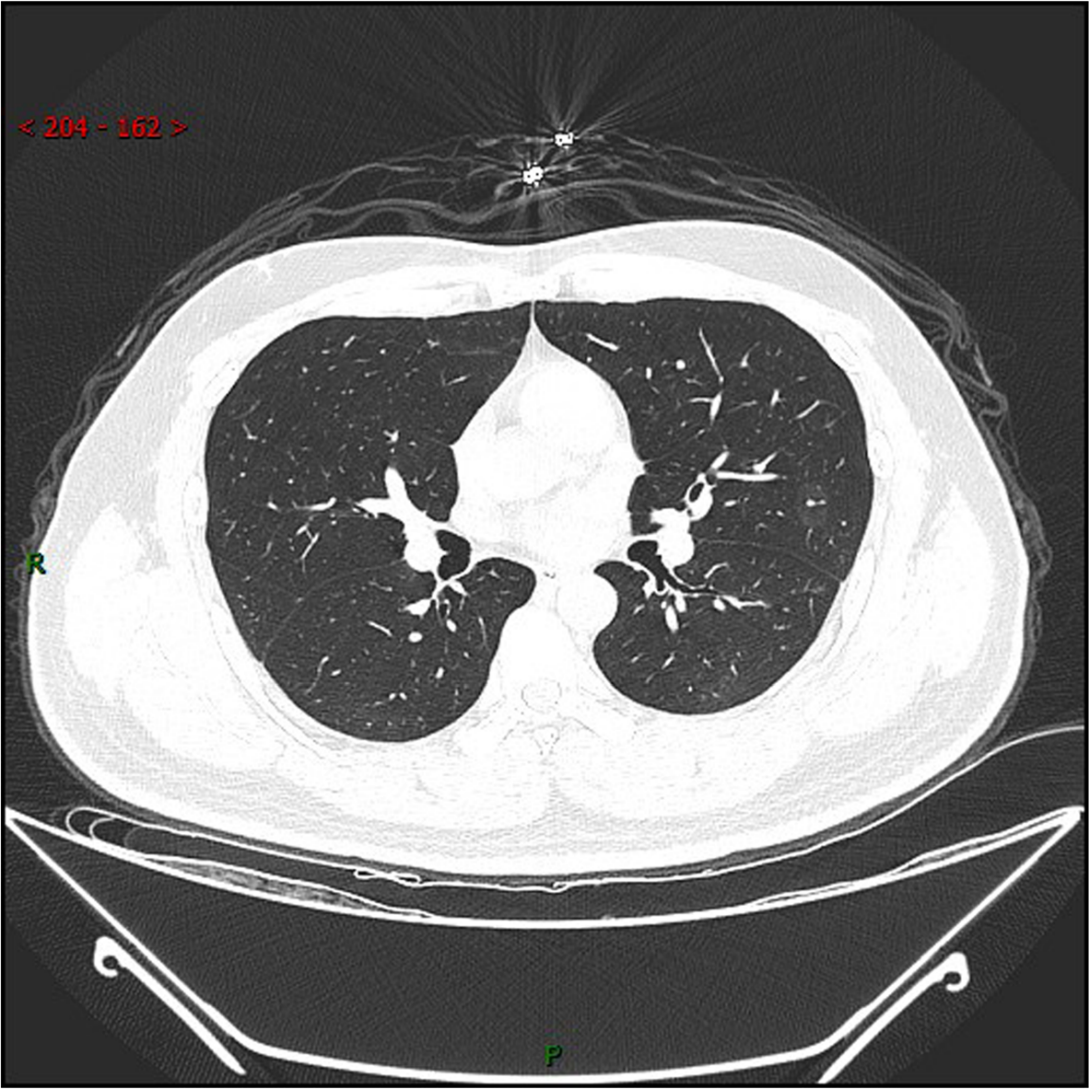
Chest CT of SARS-CoV-2 reactivation patient. A 30-year-old male patient was negative for PCR at 2 weeks’ follow-up but reverted to positive for RT-PCR at 4 weeks. The range of ground-glass opacities in the left upper lobe narrowed and the density increased slightly, while the density of ground-glass opacities in the lower right lobe decreased. R: right
